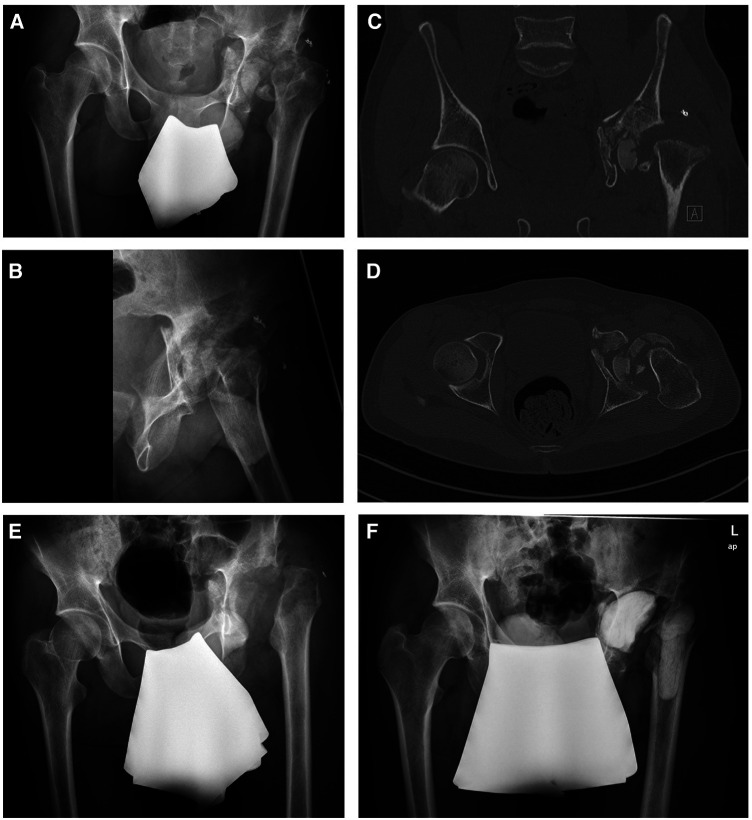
Preoperative x-rays (A,B) and CT scans showing a multi-fragmentary acetabular and femoral head fracture. After septic debridement, removal of remaining foreign bodies and removal of osseous fragments (E), an antibiotic-loaded cemented filler was implanted to the left acetabulum and proximal femur (F).
